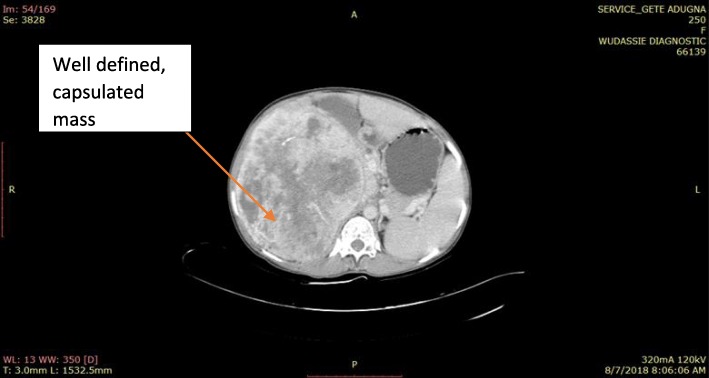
Computed tomography scan of solitary fibrous tumor of right adrenal gland of a 13-year-old girl
